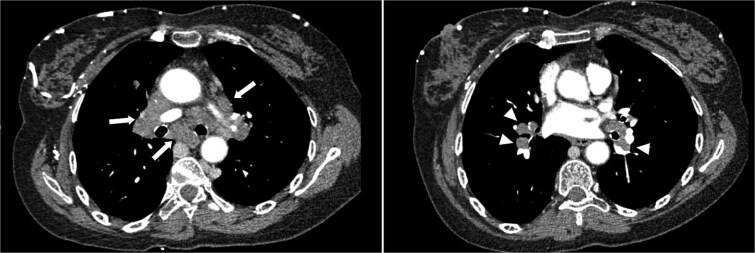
Axial CT scan showing multiple mediastinal (arrows) and hilar (arrowheads) lymphadenopathies, with no venous compression.
